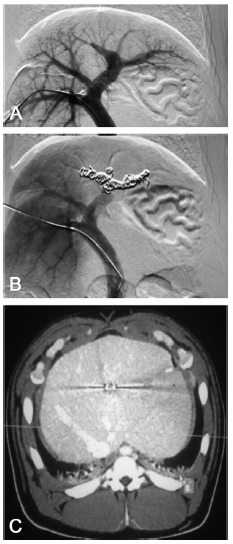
A) Left portal vein showed by portography; B) right portal vein after embolization; C) CT scan with IV contrast after portal vein embolization
